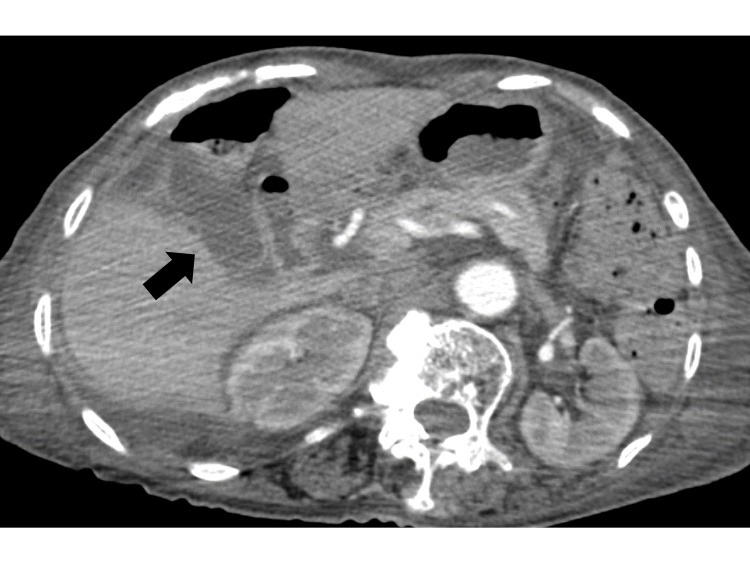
Contrast-enhanced abdominal computed tomography revealing gallbladder edema without strong wall enhancement
